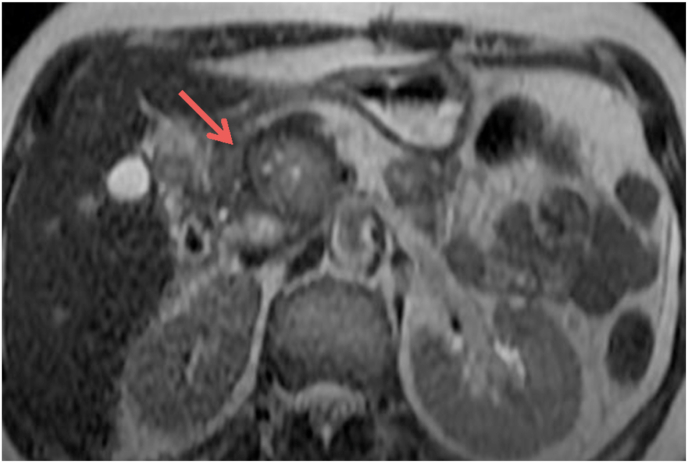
MRI a T2-weighted axial sequence showing an encapsulated mass with well-defined borders located in the pancreas's head (arrow).
